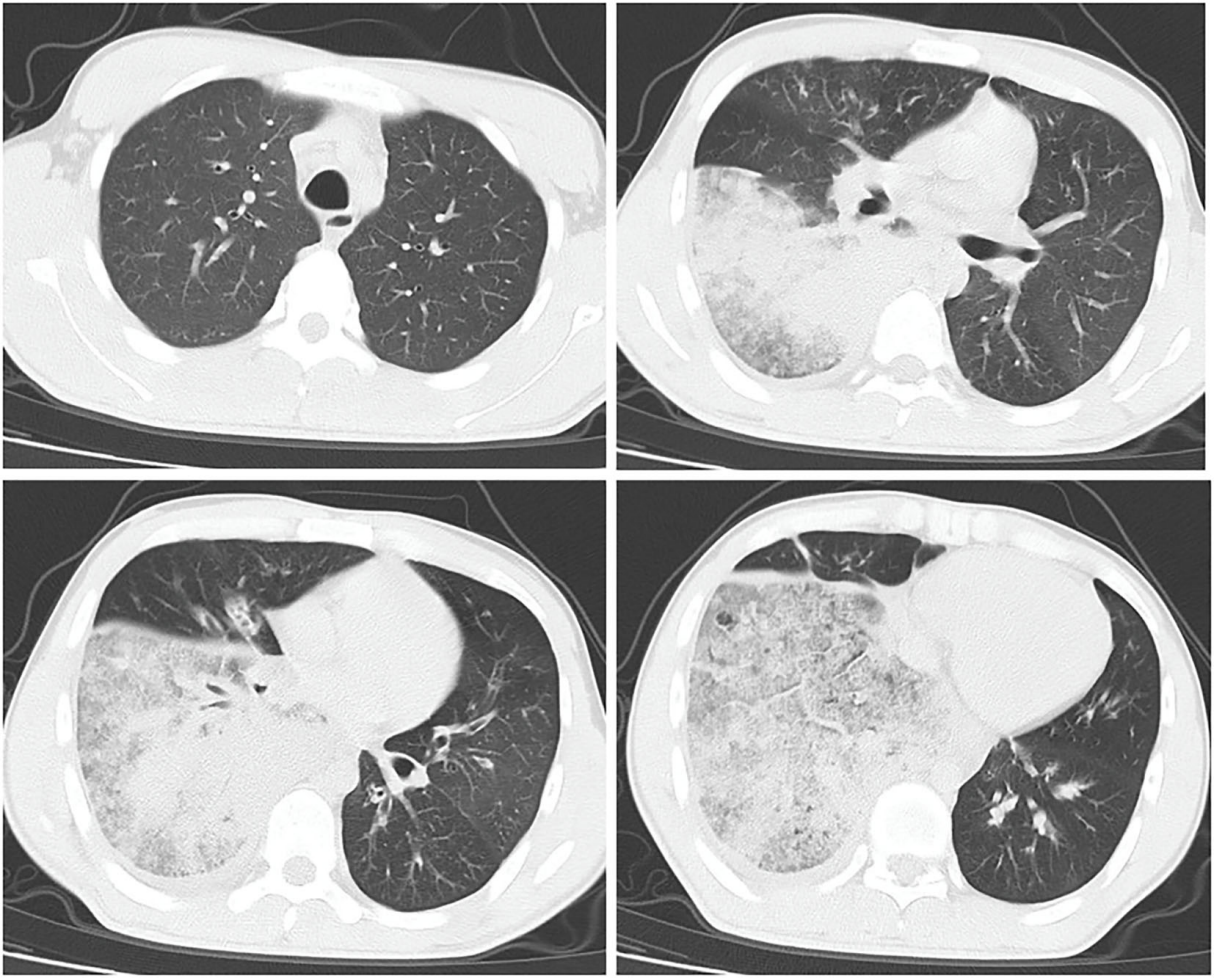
CT results of the lungs.
